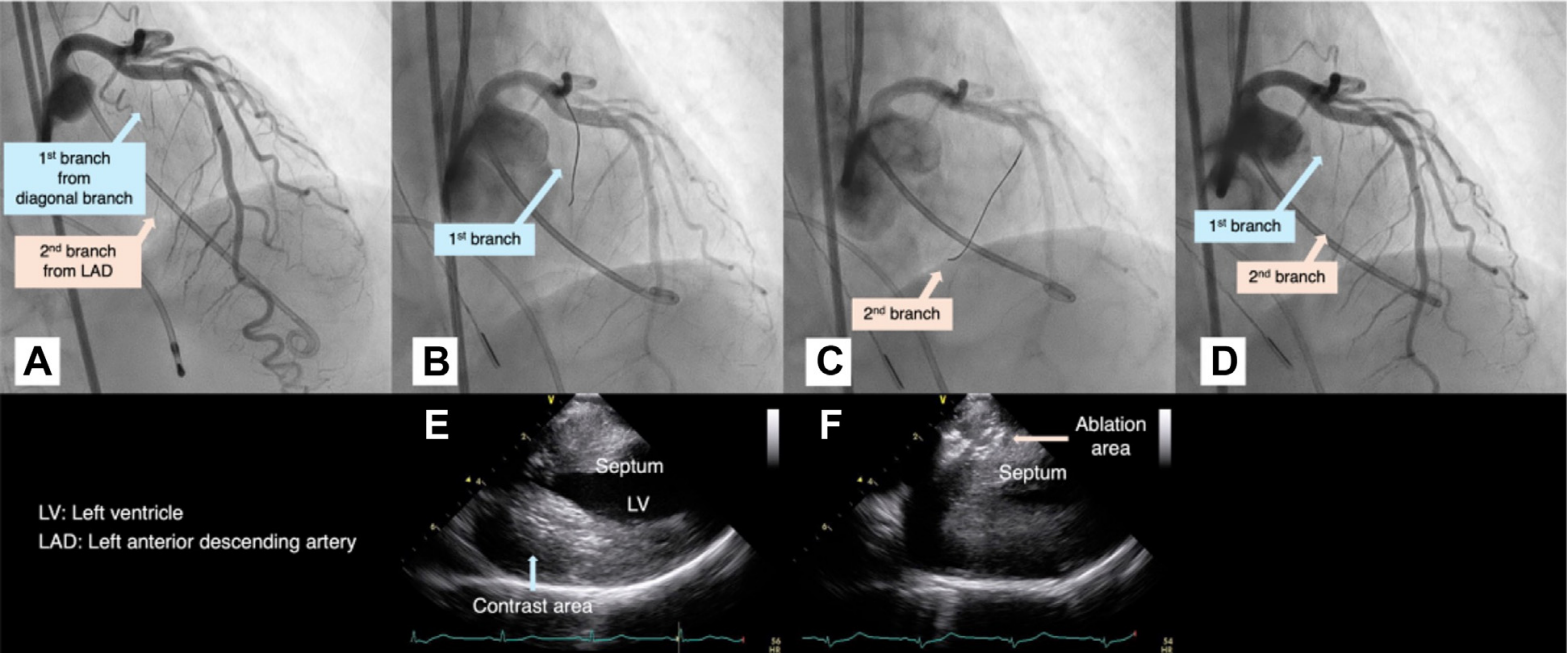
Imaging in Case 2, Second Session (A to D) Coronary angiography (A) before ablation, (B) during wiring to the first branch from the diagonal branch, (C) during wiring to the second branch from the left anterior descending (LAD) artery, and (D) post ablation. (E and F) Jugular vein intracardiac echocardiography showing (E) contrast agent injection to the first branch from the diagonal branch and (F) ethanol injection to the second branch from the left anterior descending artery. High signal intensity is observed up to the membranous septal region. LV = left ventricle.
